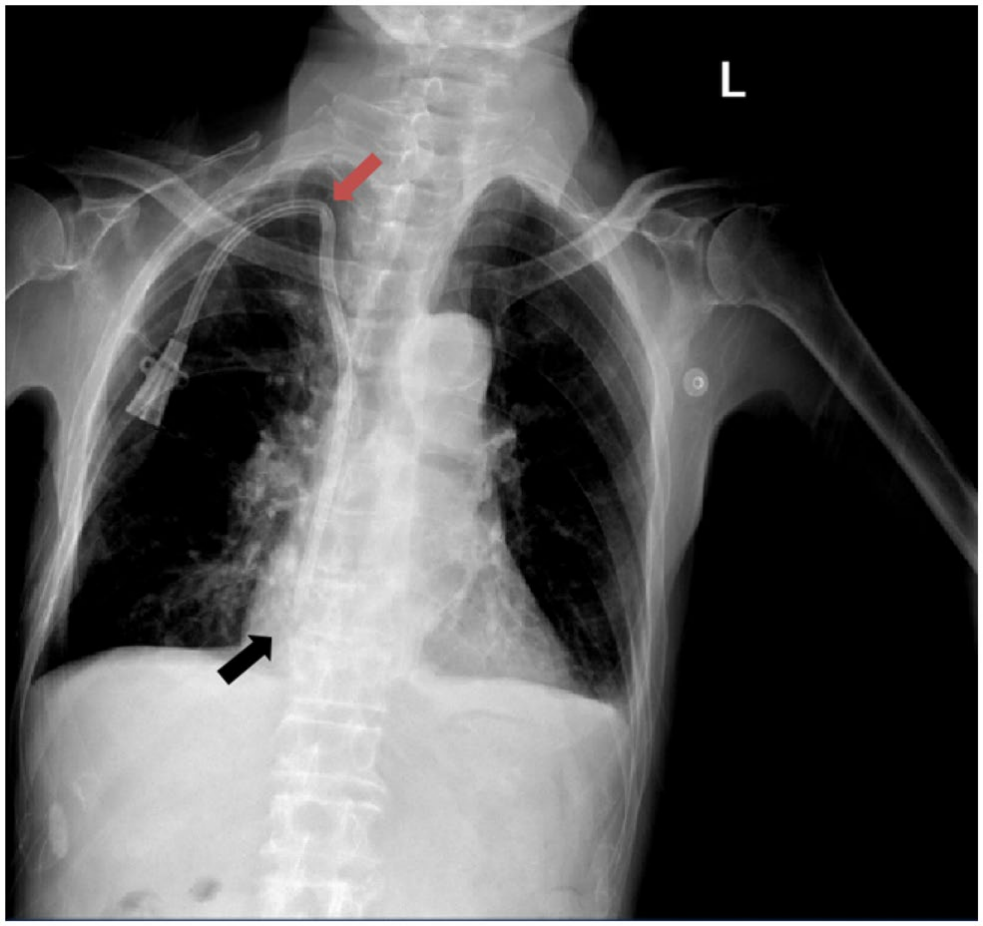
Ultrasound-guided group required postoperative chest X-rays for review. Red arrows indicate the occurrence of kinked catheter events, while the black arrows indicate the position of the catheter tip.
